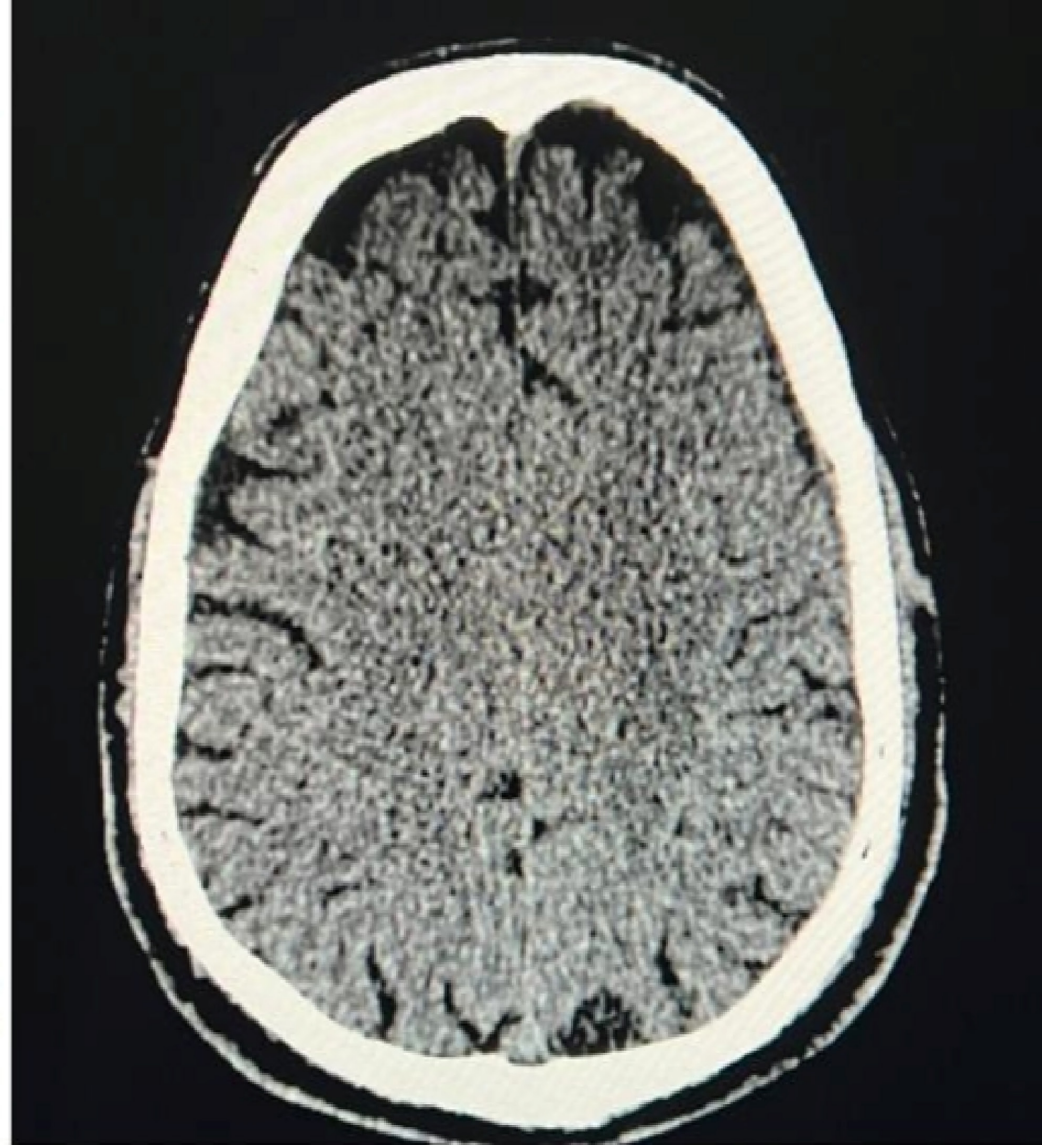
CT scan showing resolution of hematoma after embolization of the middle meningeal artery.
